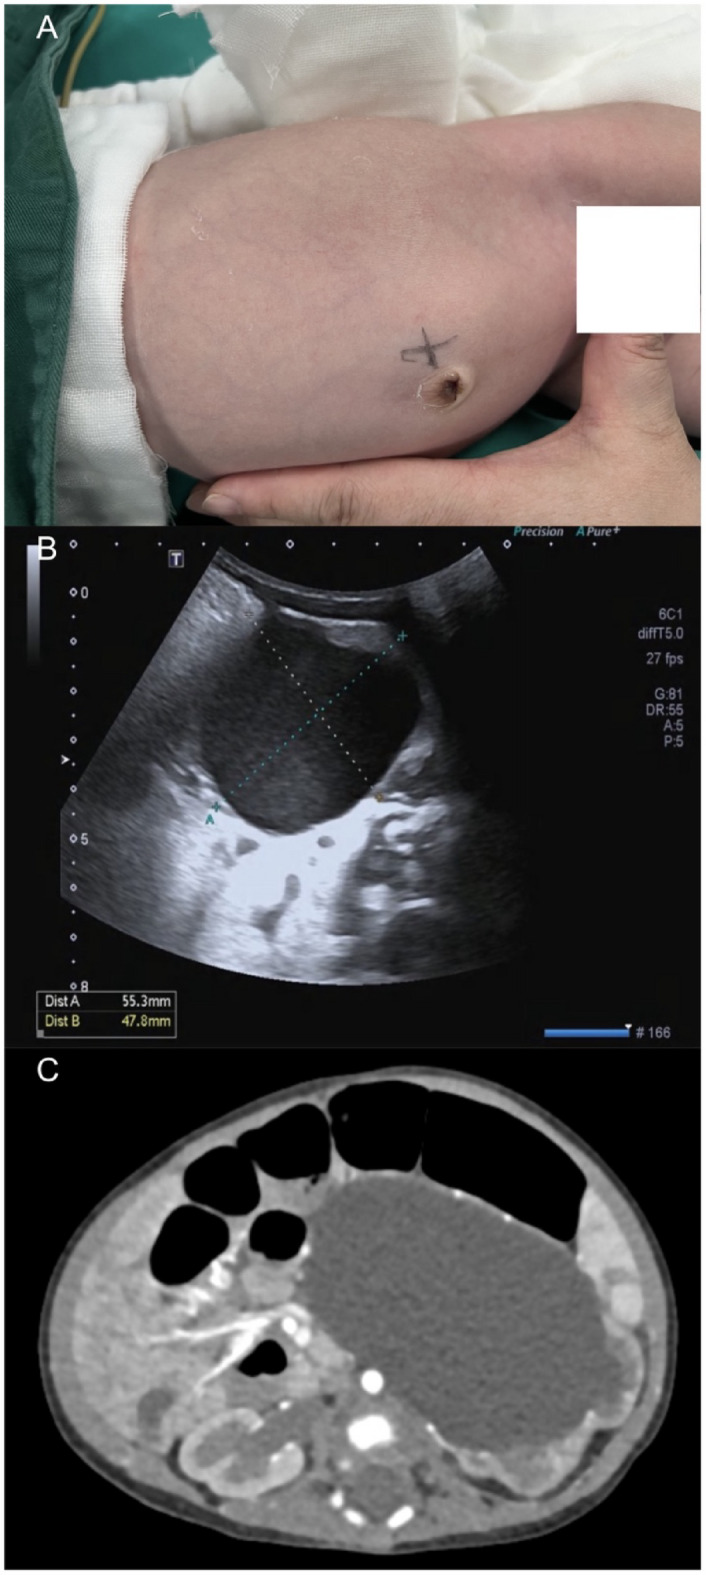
(A) The diagnosis presented hydronephrosis (B) Preoperative US showed hydronephrosis (C) Outcomes of preoperative CT indicated severe hydronephrosis.
